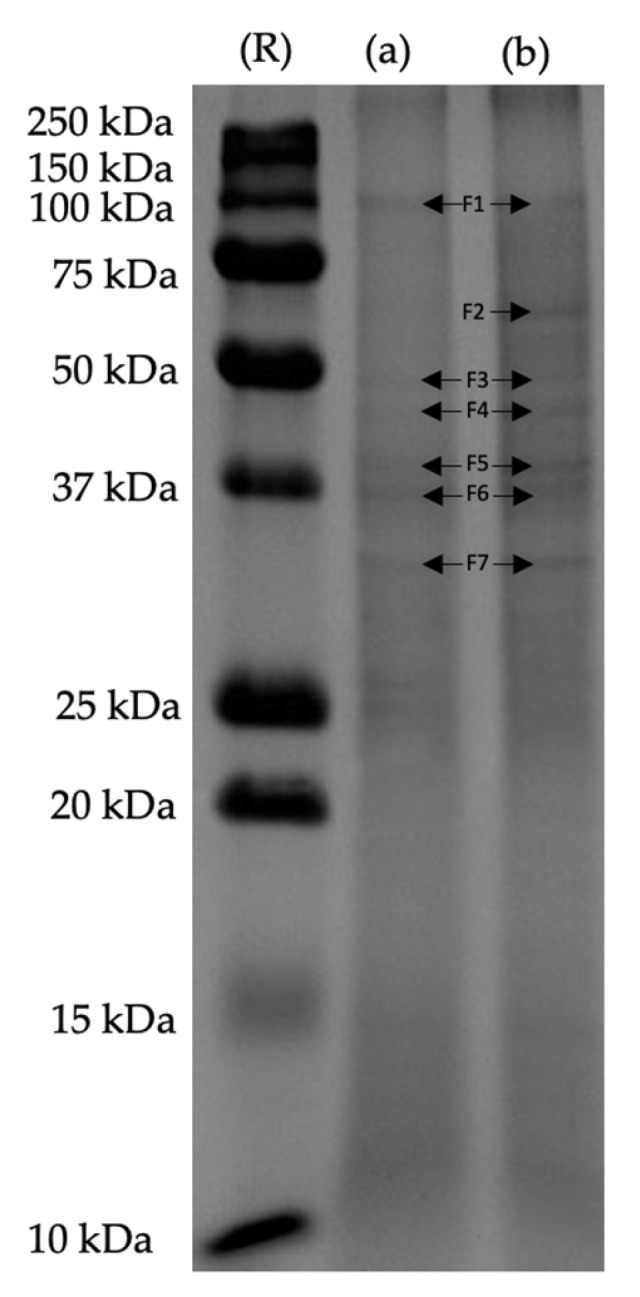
Molecular weight profile of the (a) control and (b) ultrasound protein extracts from duckweed by SDS-PAGE. Reference (R); 100 KDa fraction (F1); 63 KDa fraction (F2); 50 KDa fraction (F3); 45 KDa fraction (F4); 39 KDa fraction (F5); 37 KDa fraction (F6); and 32 KDa fraction (F7).
